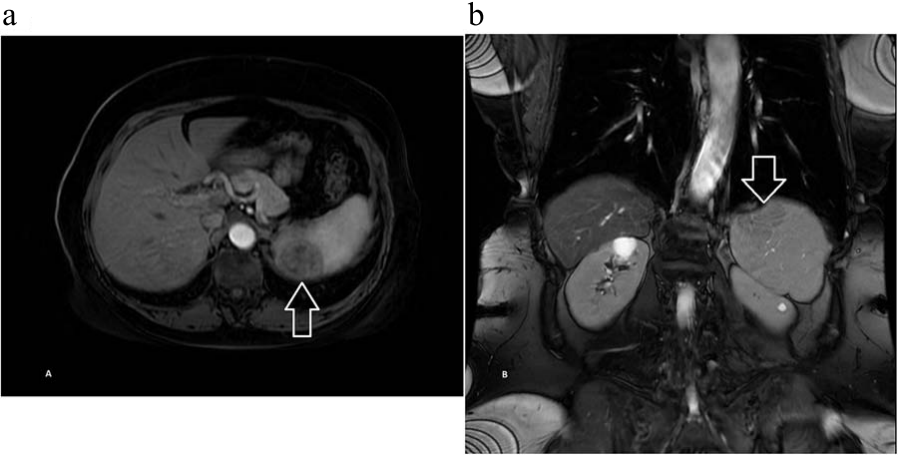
Magnetic resonance imaging of the abdomen with contrast showing a well-circumscribed 3.7 × 3.7 cm solid lesion in the lower pole of the spleen with a decreased signal on T2 on (a) axial and (b) coronal views with arrow marking the mass.
